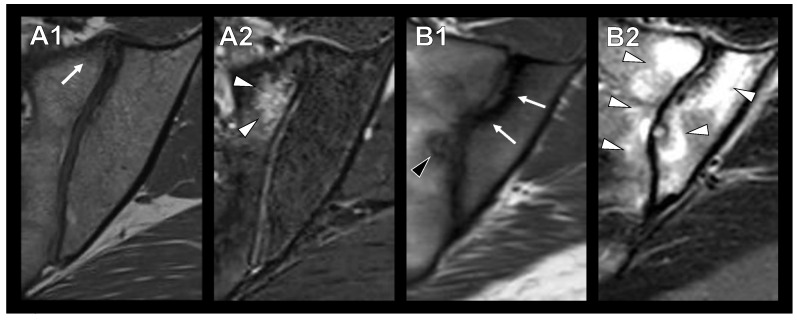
Appearance of OCI and sacroiliitis in axial spondyloarthritis in axial T1-weighted (A1,B1) and T2-STIR (A2,B2) MR images. A1/A2: Patient with OCI and anterior sclerosis (A1, white arrow) and bone marrow edema (A2, white arrowheads). B1/B2: Patient with sacroiliitis with randomly distributed sclerosis (B1, white arrows) and bone marrow edema (B2, white arrowheads) as well as erosions (B1, black arrowhead).
